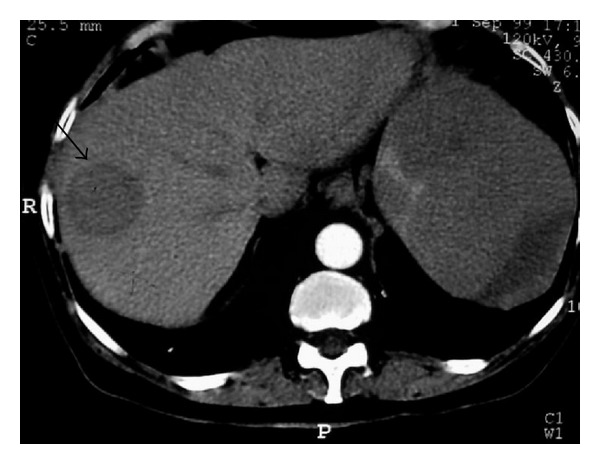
A 40-year-old woman with lymphoblastic lymphoma. Axial contrast-enhanced abdominal CT demonstrates circumscribed low-attenuation mass in the liver (arrow). Hypodense lesions are also seen in spleen.
